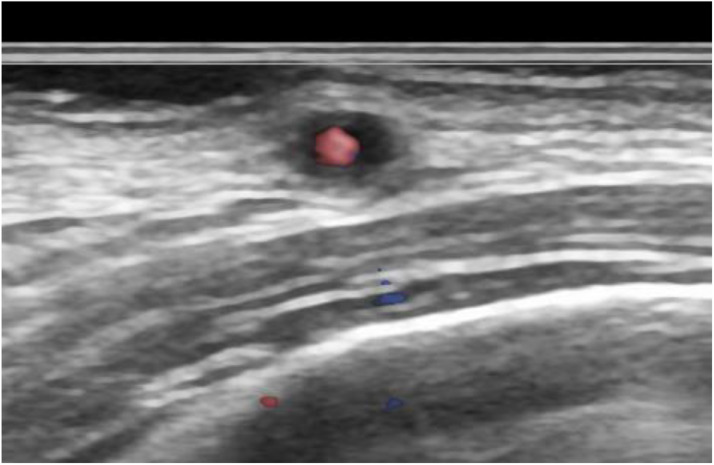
Transverse view of color Doppler ultrasonography depicting the superficial temporal artery. A “halo sign,” consisting of a uniformly hypoechoic thickened wall encircling residual color flow, is visible.
